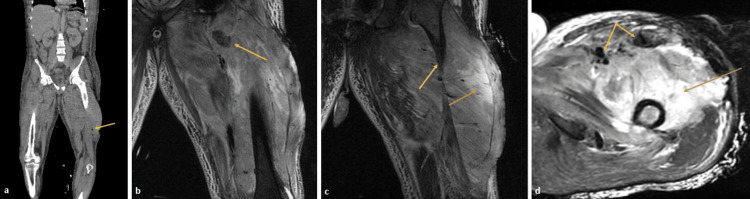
Imaging consistent with myofasciitis and diffuse myositis (a) Small pockets of air seen in distal posterior thigh (yellow arrow) on coronal view of CT left lower extremity. (b) MRI showing a focal area with low T2 and high T1 signal intensity within the iliopsoas muscle measuring approximately 2.2 x 1.9 x 2.8 cm suspected to reflect an area of focal myonecrosis (yellow arrow). (c) Diffuse subcutaneous soft tissue edema (orange arrow) with small ill-defined fluid collections (yellow arrow) within the deep subcutaneous soft tissues adjacent to the fasciotomy site. (d) Diffuse subcutaneous soft tissue edema (orange arrow) with small ill-defined fluid collections (yellow arrow) within the deep subcutaneous soft tissues adjacent to the fasciotomy site.
